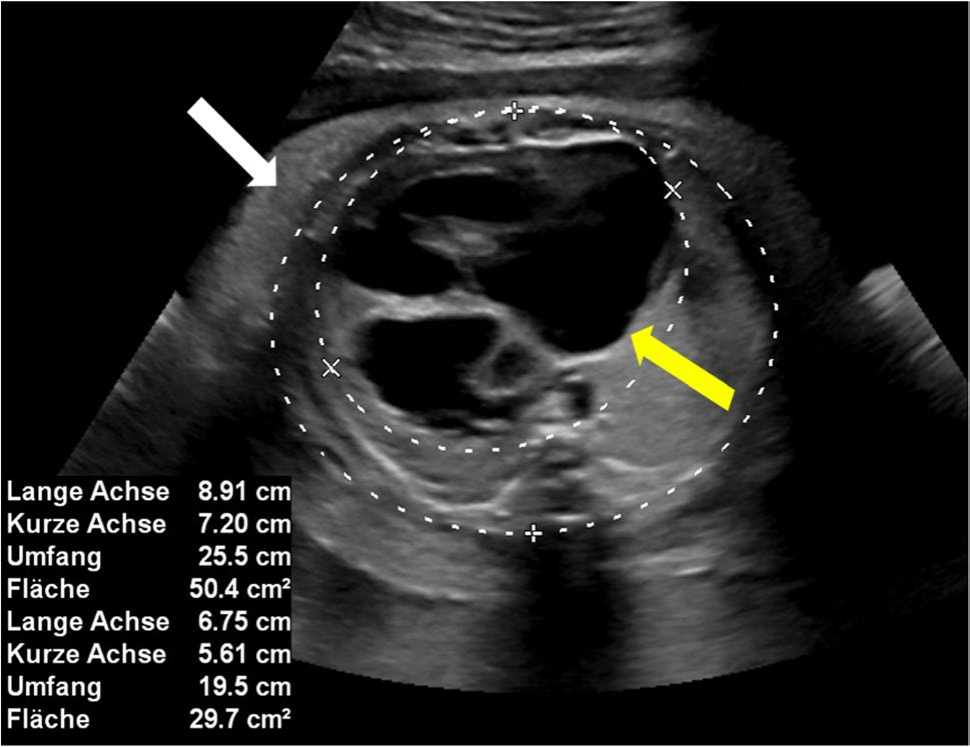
Case 3 at 23 + 4 weeks of gestation with severe cardiomegaly, CVPS 4 points, (ascites, pleural effusions: 1 point, severe cardiomegaly (cardiothoracic area ratio = 0.59): 0 points, tricuspid valve regurgitation: 1 point, normal arterial Doppler flow velocimetry: 2 points, pulsatile umbilical vein: 0 points), yellow arrow: heart, white arrow: skin edema
